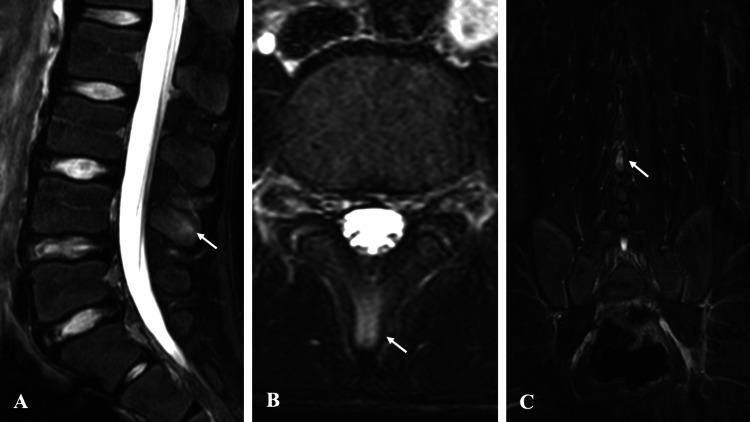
Pre-treatment MRI findings in Case 2. STIR sequence MRI reveals high signal intensity at the cranial aspect of the L4 spinous process: (A) sagittal view, (B) axial view, and (C) coronal view. MRI, magnetic resonance imaging; STIR, short-tau inversion recovery
